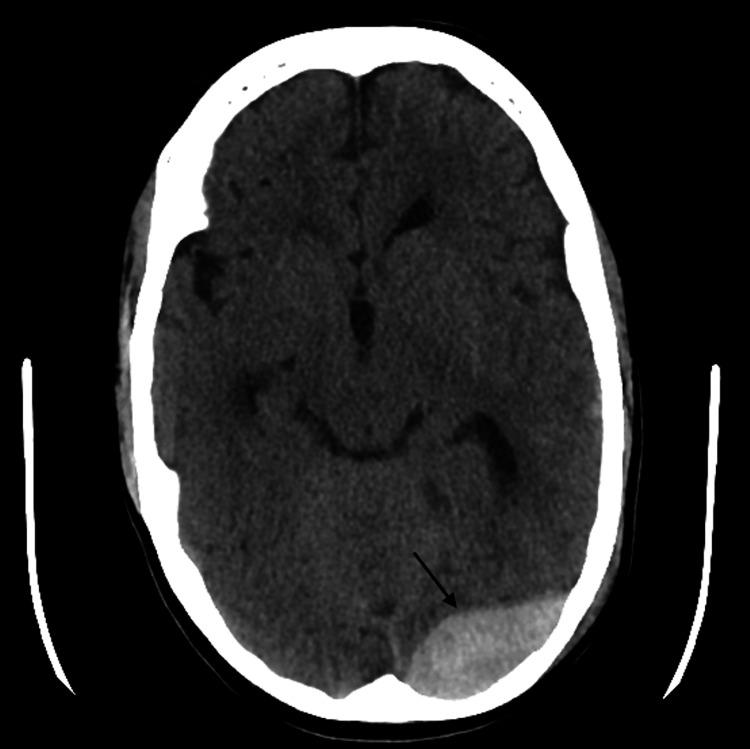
Preoperative axial CT scan showing the location of the epidural hematoma (black arrow), without significant midline deviation
